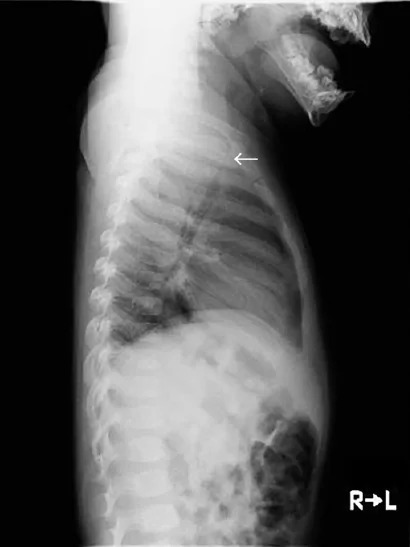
Lateral plain chest radiograph showing tracheal stenosis in a toddler who presented with acute-onset dyspnea (arrow).
